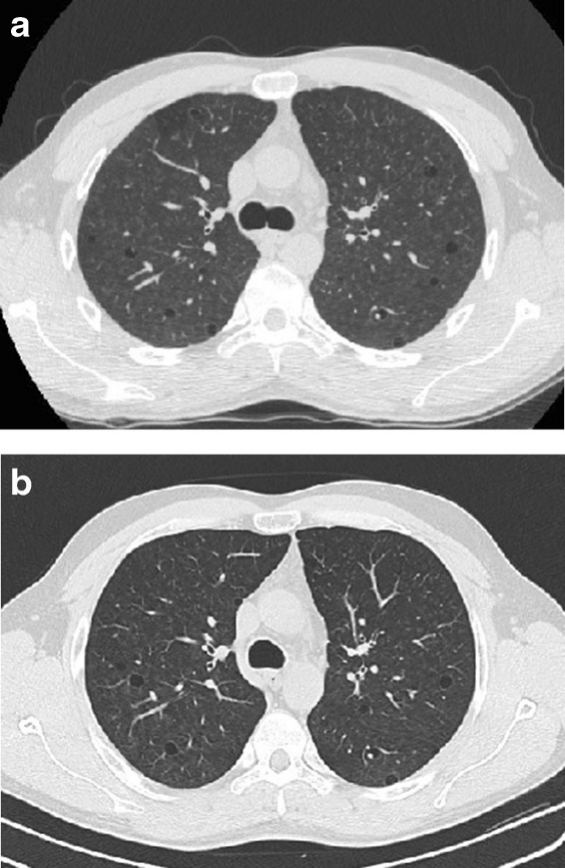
A 35-year-old man, smoker, with asymptomatic PLCH (chest radiograph performed for traumatic injury). Diagnosis based on typical lesion heterogeneity on CT imaging. a Two-millimetre collimated CT images at initial diagnosis shows bilateral centrilobular ground-glass nodules, thin-walled cysts and a single air-trapping zone. Upper and middle lung are predominantly affected. b Three-millimetre collimated CT after 5 months of smoking cessation shows almost complete resolution of the centrilobular nodularity while thin-walled cysts remain unchanged
